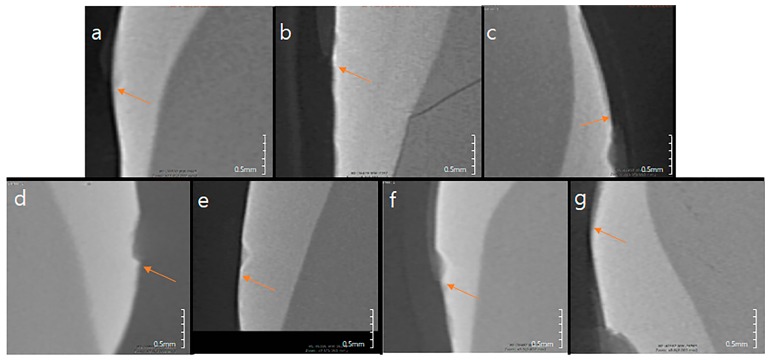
Remineralization point of the BAG and ion (silver or zinc)-doped BAG orthodontic bonding primer via CBCT. (a) Control; (b) BAG 0.2%; (c) BAG 1.0%; (d) BAG@Ag1 0.2%; (e) BAG@Ag1 1.0%; (f) BAG@Zn5 0.2%; and (g) BAG@Zn5 1.0%.
